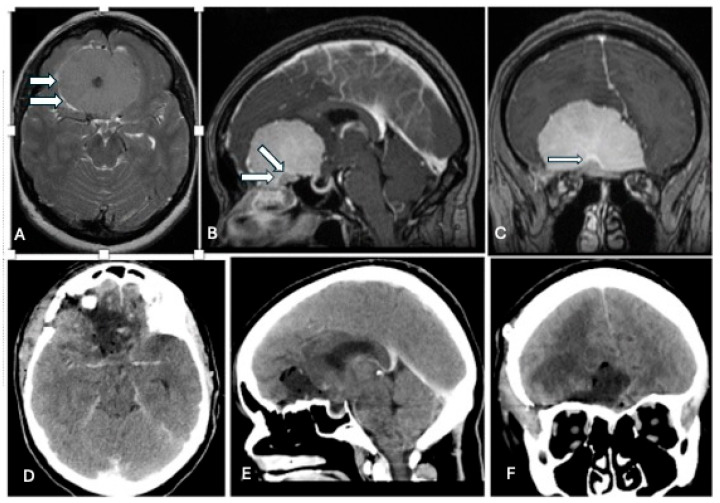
A preoperative CE-T1WI and postoperative CT of a large ophthalmic groove meningioma (A–C), which was completely resected via a right frontolateral approach (D–F). Note the presence of an arachnoidal cleavage plane (image (A) arrow) and dural enhancement at the level of the ophthalmic groove (images (B,C), arrows).
